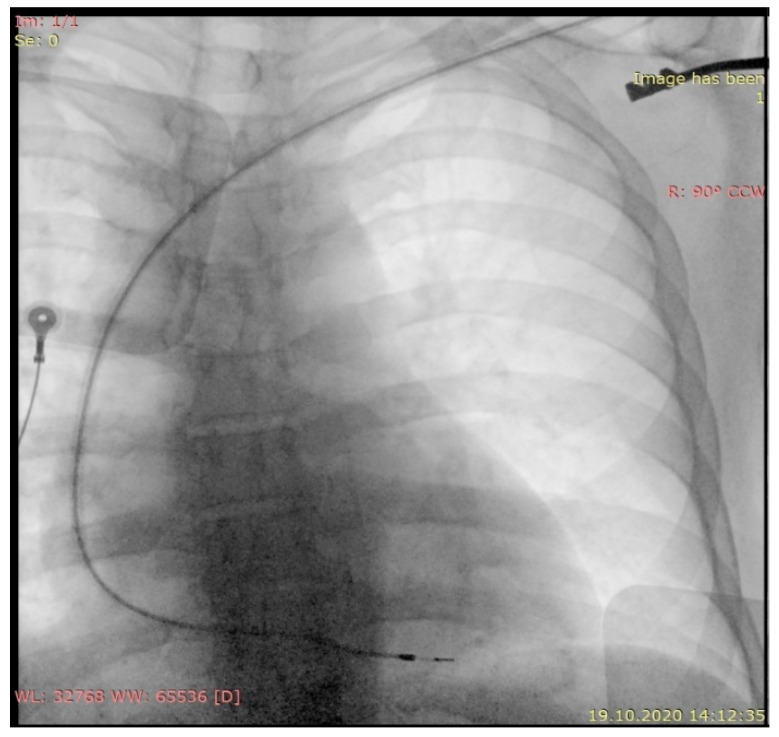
Antero-posterior chest X-ray: passive fixation lead on the topography of the right ventricle.
